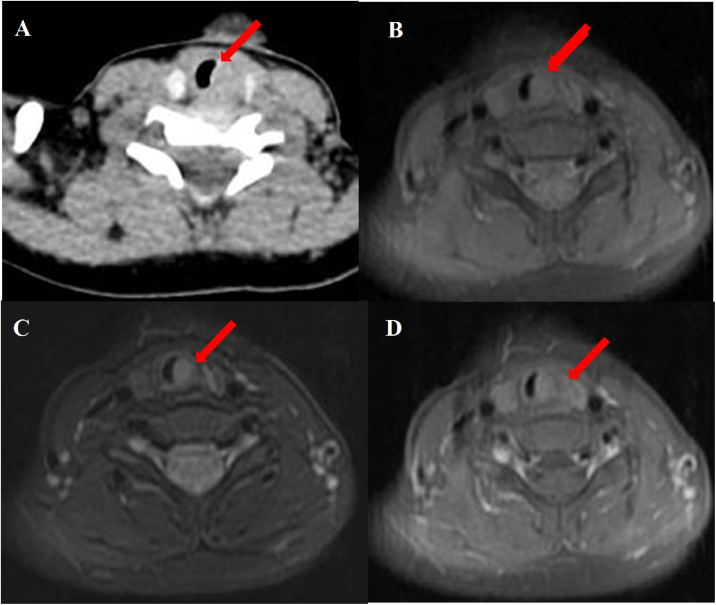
Subglottic masses in the 2-year-old boy presenting with stridor. Computed Tomography (CT) and Magnetic Resonance Imaging (MRI) scan of the neck showed (A) an abnormal soft-tissue density shadow on the medial side of bilateral thyroid upper pole with corresponding slightly narrowed airway (arrow). The thymus shadow was larger and seemed to extend to the neck. Transverse view of T1-weighted and Iterative Decomposition of water and fat with Echo Asymmetric and Least-squares estimation (IDEAL) T2-weighted MRI showed (B) isointense T1 and (C) hyperintense T2 masses in left of the trachea at the level of the thyroid gland and at the edge of the left and right lobe of the thyroid gland. (D) The masses enhanced slightly in contrast-T1-weighted transverse view. The trachea (arrow) was locally narrowed at the level of the thyroid gland.
